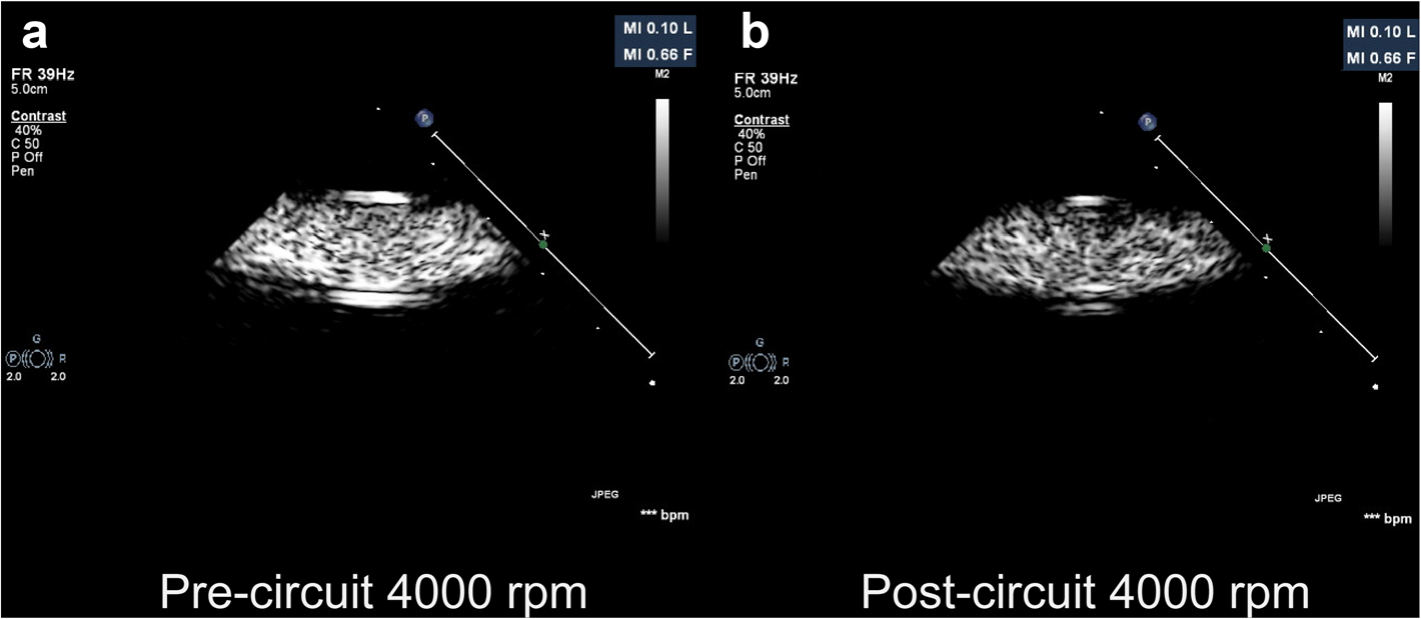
a, b Pre-ECMO and post-ECMO contrast echocardiographic images. Note the reduced signal intensity in the post-ECMO image
